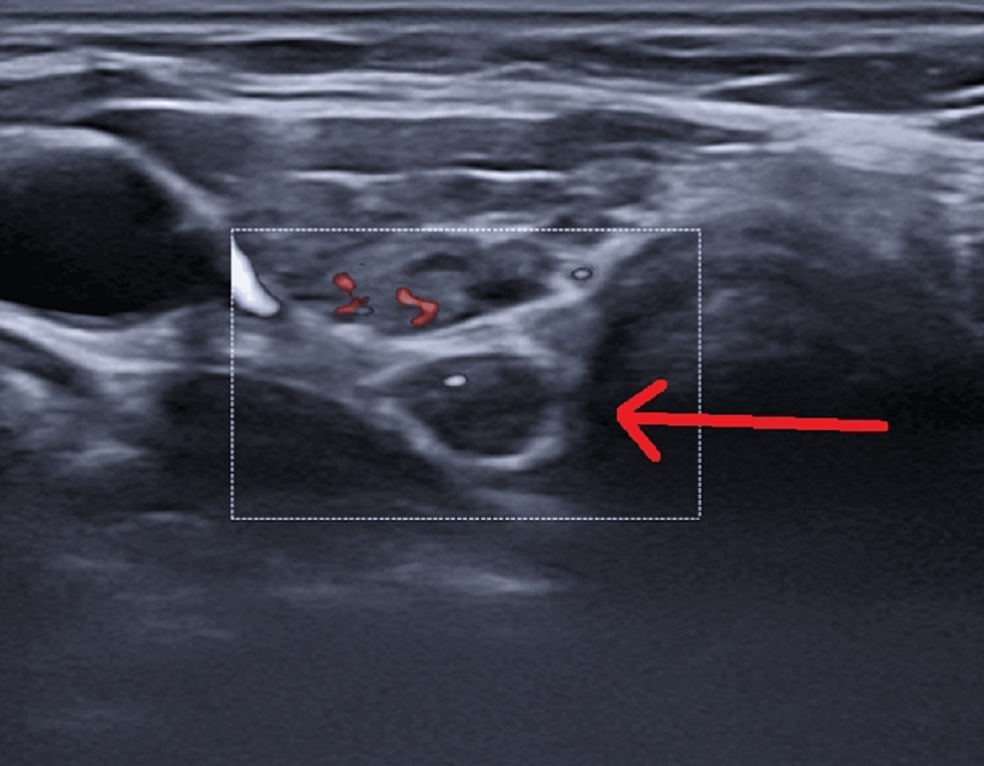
Ultrasound imaging. The arrow shows a parathyroid adenoma under the lower pole of the right thyroid lobe-hypoechoic formation with sharp and smooth outlines and increased blood flow.
